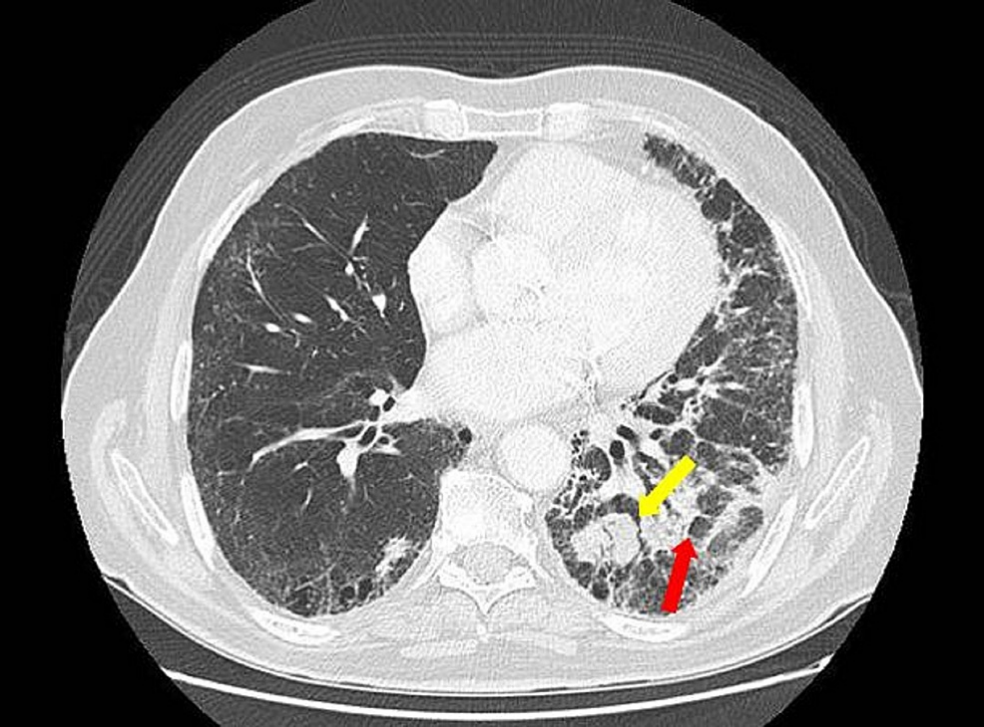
CT showing regression of the pulmonary tumor, presence of multiple bilateral mediastinal and hilar adenopathies, as well as fibrotic changes in the left posterior-basilar lung The yellow arrow is showing the mass; the red arrow is showing fibrosis of the parenchyma.
